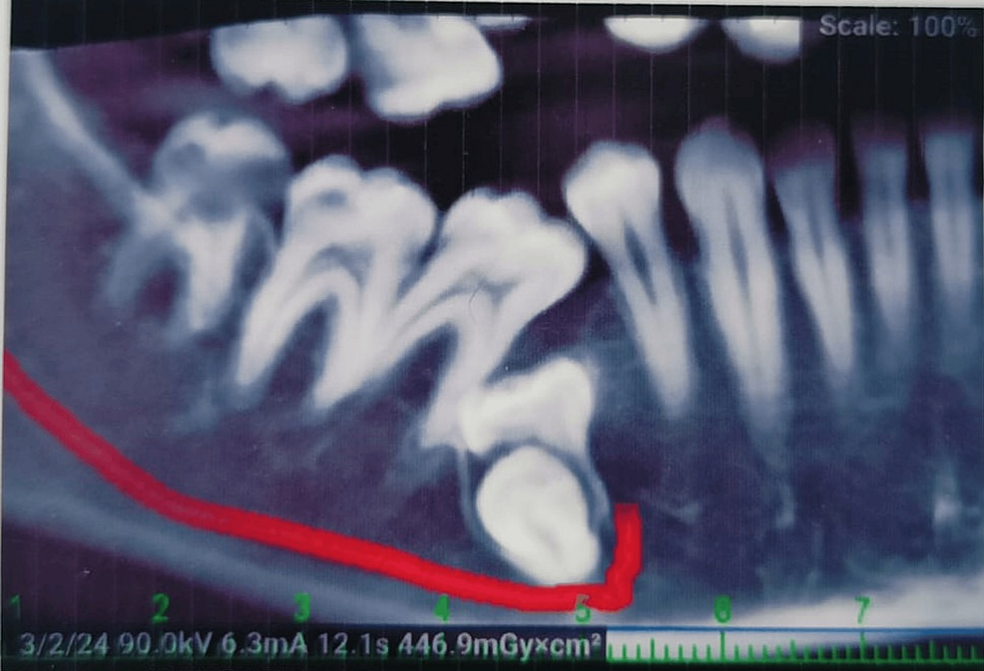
Radiograph of the impacted site
